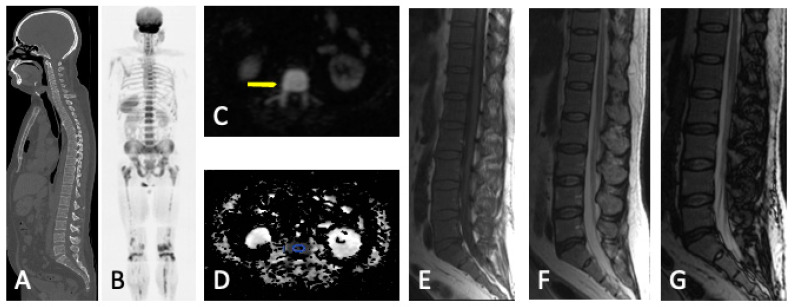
Diffuse MM bone marrow involvement of the axial and peripheral skeleton. No visible lytic lesions in WBLDCT (A). Diffuse bone marrow infiltration with high SI in b-900 MR-DWI ((B) and arrow in (C)) and pathological ADC values (878 μm^2^/s) in the vertebral body (circle in (D)). Malignant imaging features with diffuse bone marrow hypointensity in T1WI (E) and an SI drop of <20% between the in-phase (F) and opposed-phase MRI (G).
